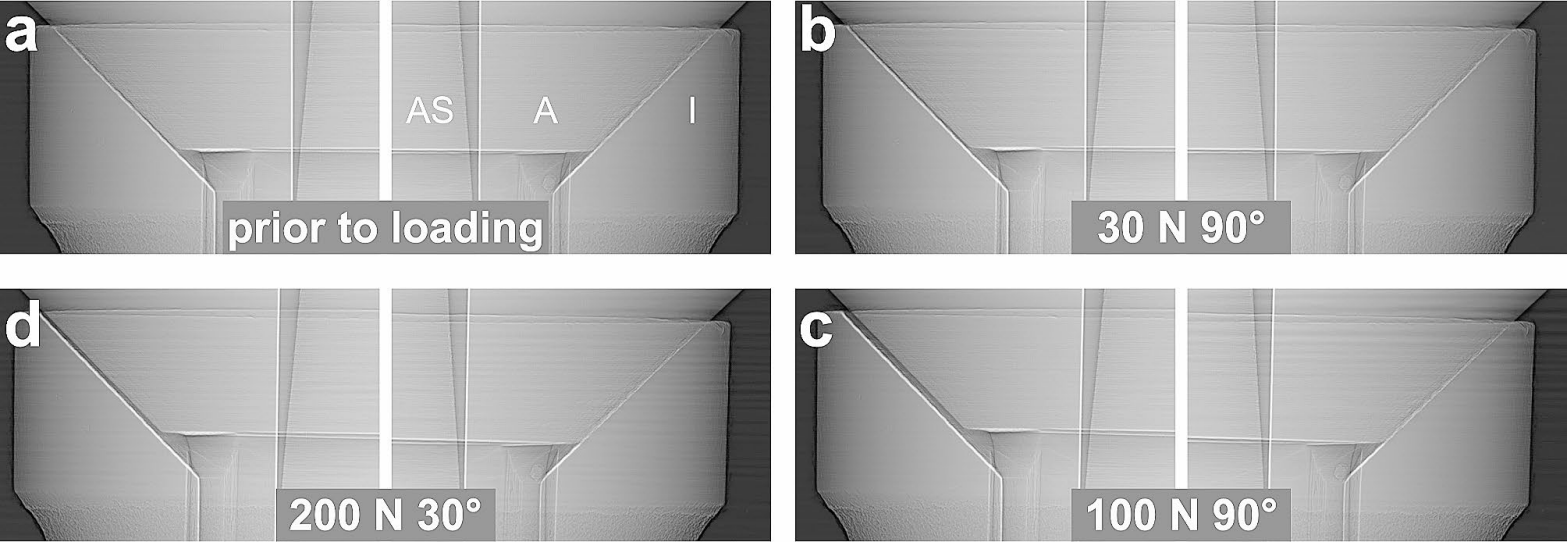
Phase-contrast radiographies of BE butt-joint connection prior to loading (a) and at force application of 30 N at 90° (b), 100 N at 90° (c) and 200 N at 30° (d) from the left side. Abutment displacement leads to almost parallel gap formation. Despite large microgaps (up to 40.5 μm) no implant wall deformation is visible on the opposing side indicated by transparent vertical positioning lines
